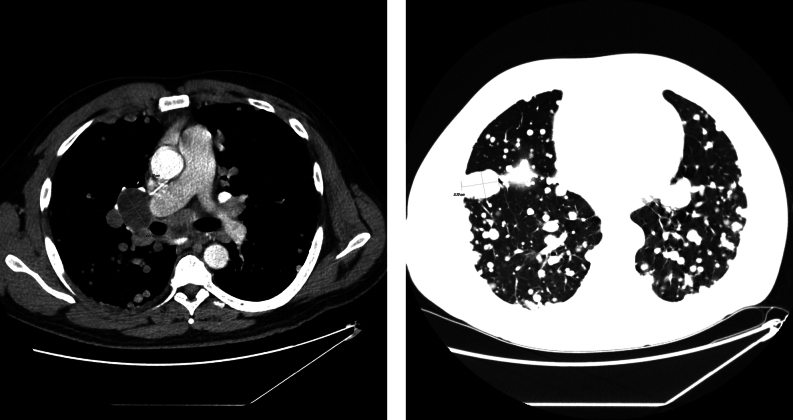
CT of the chest, performed after the administration of intravenous contrast material, revealed enlarging bilateral hilar lymphadenopathy, up to 2.6 × 3.5 cm on the right, and innumerable pulmonary nodules, up to 2.9 × 2.1 cm on the right mid lobe.
